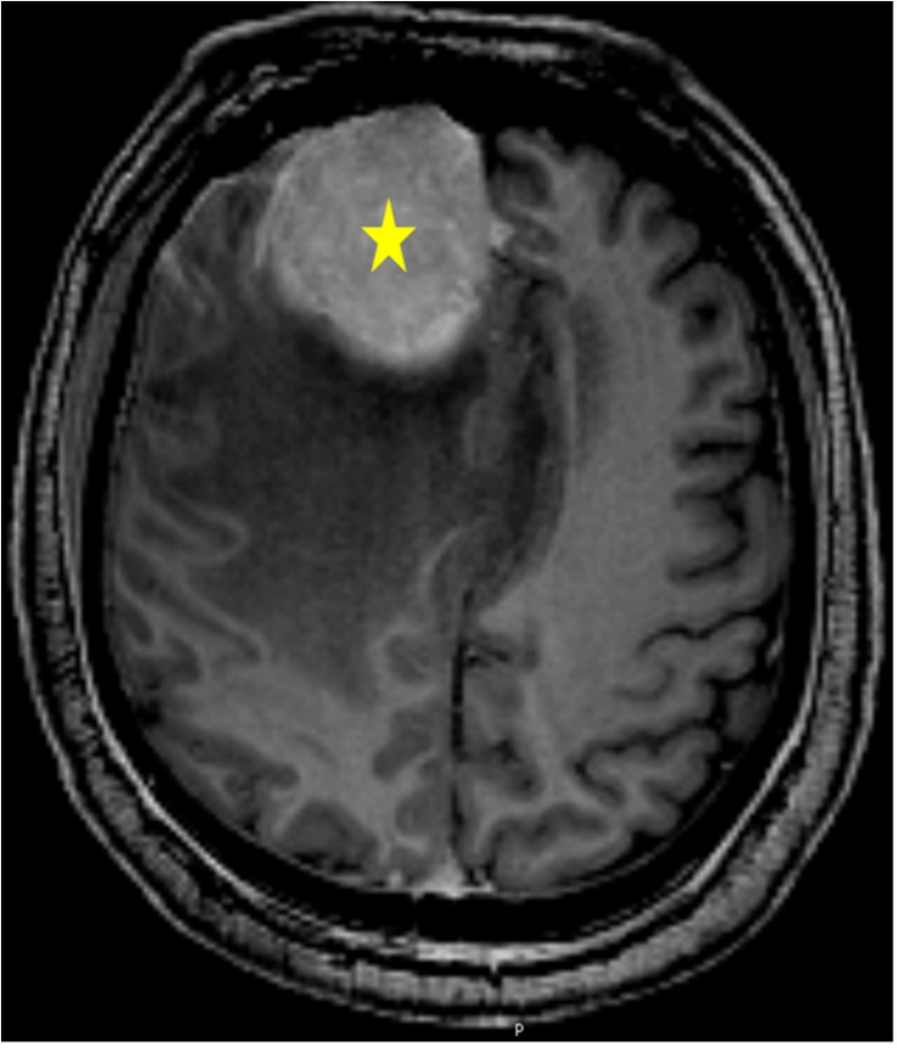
Axial Contrasted MRI MRI enhancing right parafalince extra-axial lesion (asterix) with surrounding edema and mass effect.
